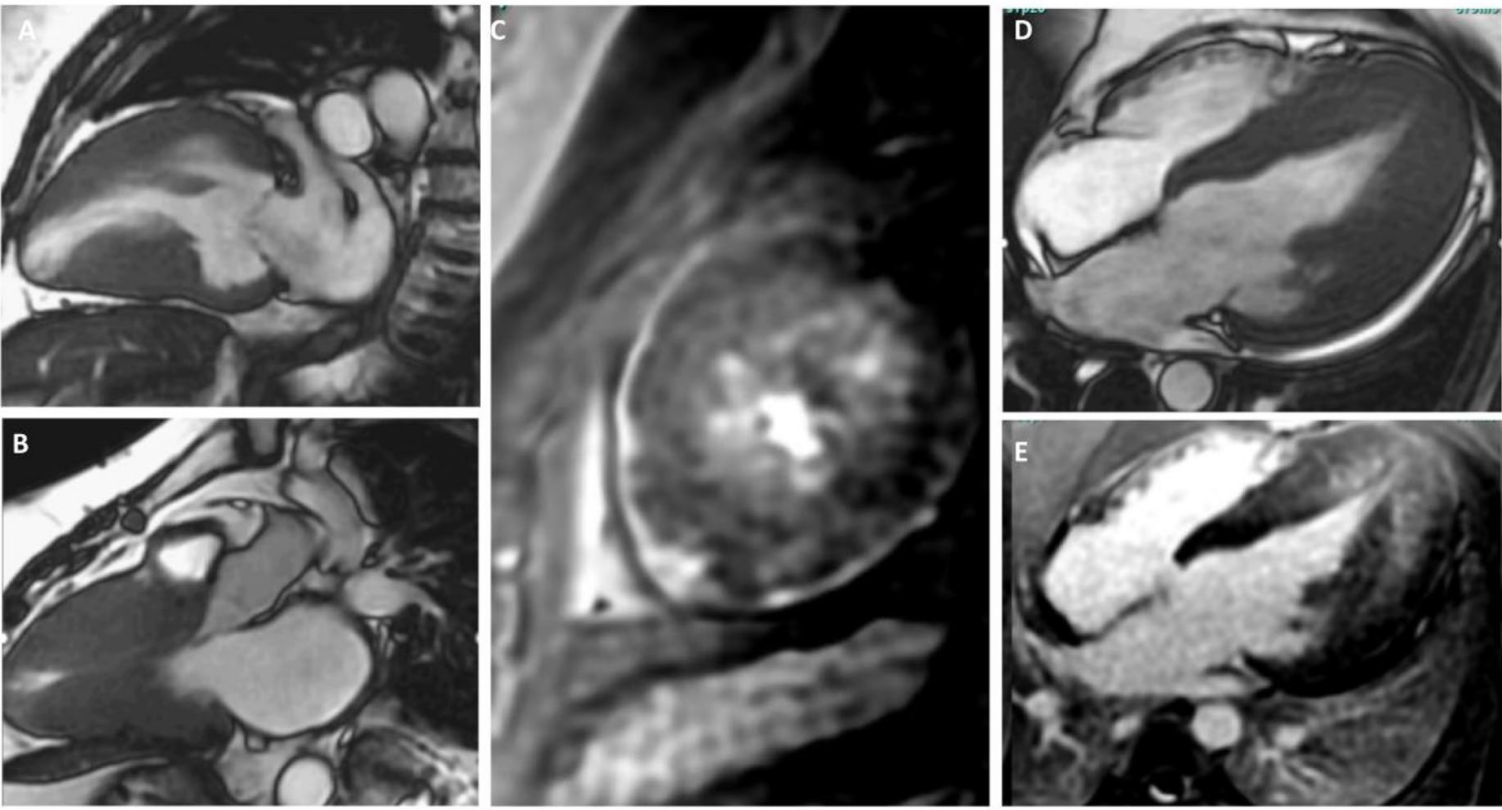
Cardiac MRI Cine SSFP images showing 2,3, and 4-chamber views
